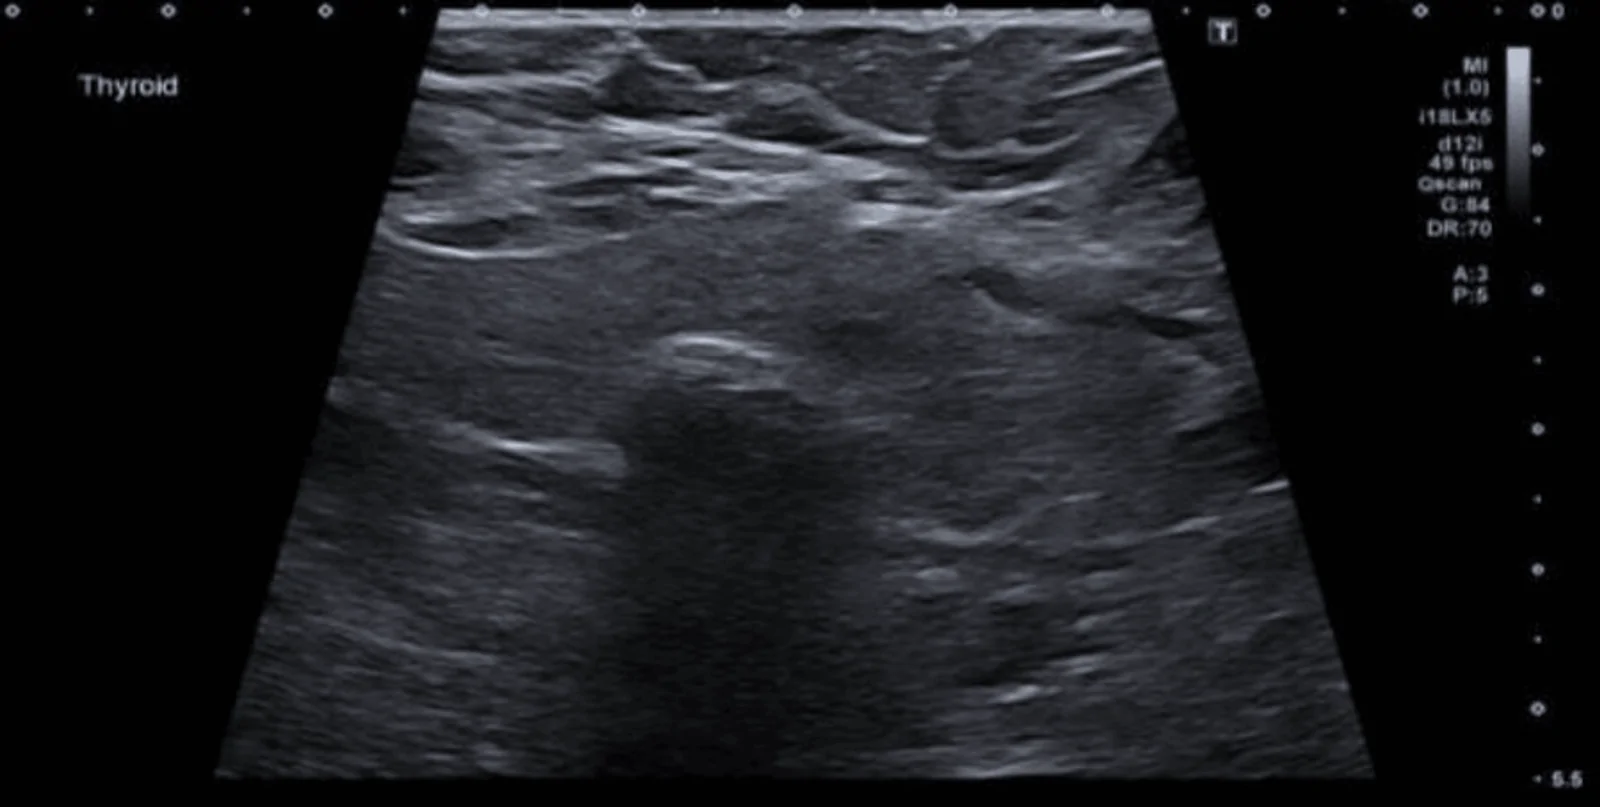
Thyroid ultrasound scan, reported as unremarkable
